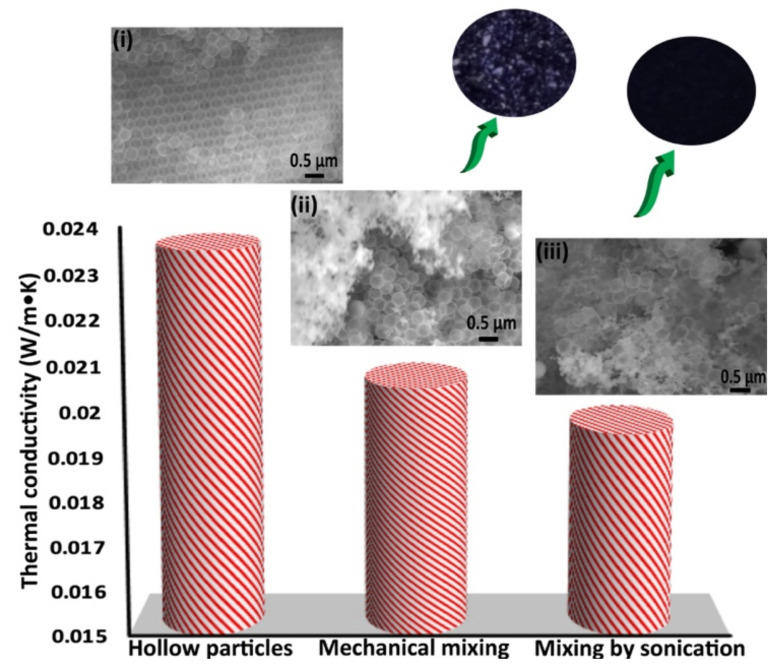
A plot showing the effect of CB dispersion on the thermal conductivity of a hollow particle–carbon black mixture. Insets (i), (ii), and (iii) are SEM images of hollow particles alone, a mechanically mixed hollow particle–CB mixture, and an ultrasonically mixed hollow particle–CB mixture, respectively. Sub-insets: Photos of mechanically and ultrasonically mixed CB and hollow particles.
